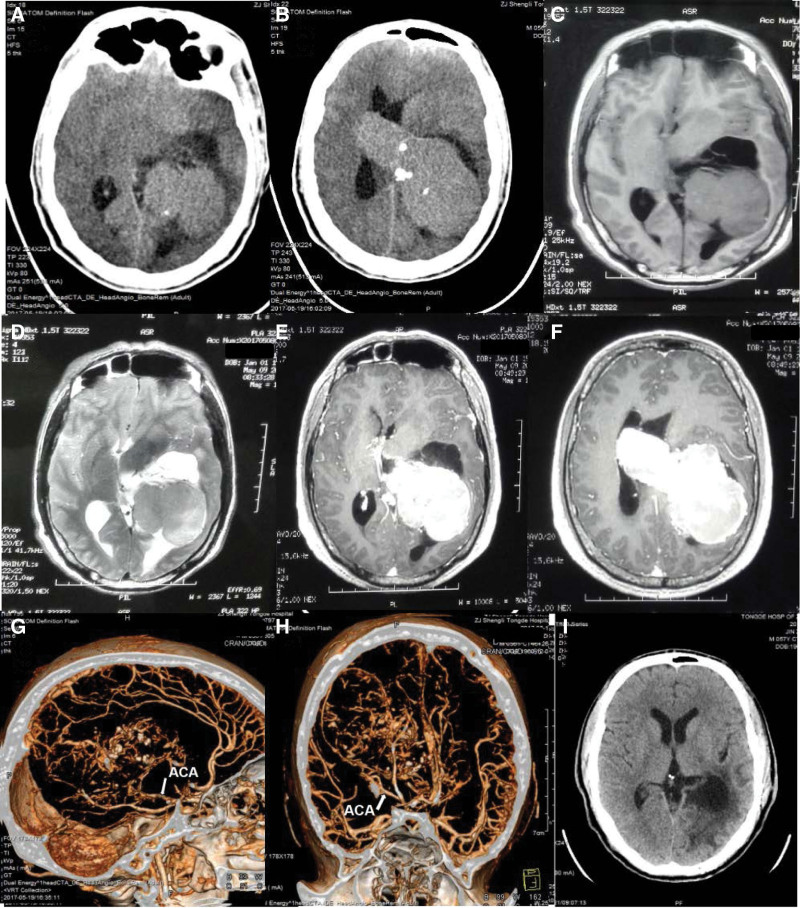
(A–I) Radiographic features of case 1 in the perioperative period. (A and B) CT scans revealed a super size heterogeneously irregular mass in the bilateral ventricle with peritumoral low-density cystic lesions. (C and D) Noncontrast-enhanced MRI scans showed solid part of the tumor was isointense on T_1_WIs, slightly hyperintense on T_2_WIs, and the cystic portion of the tumor was hypointense on T_1_WIs and hyperintense on T_2_WIs. (E and F) Contrast-enhanced T_1_WIs suggested significant enhancement of tumoral parenchyma, but non-enhanced of cyst wall. (G and H) CTA indicated an affluent blood supply of the tumoral parenchyma stemming from ACA. (I) Postoperative CT demonstrated total resection of the tumor. ACA = anterior choroidal artery, CT = computed tomography, CTA = computed tomographic angiography, MRI = magnetic resonance imaging, T_1_WIs = T_1_ weighted sequence, T_2_WIs = T_2_ weighted sequence.
